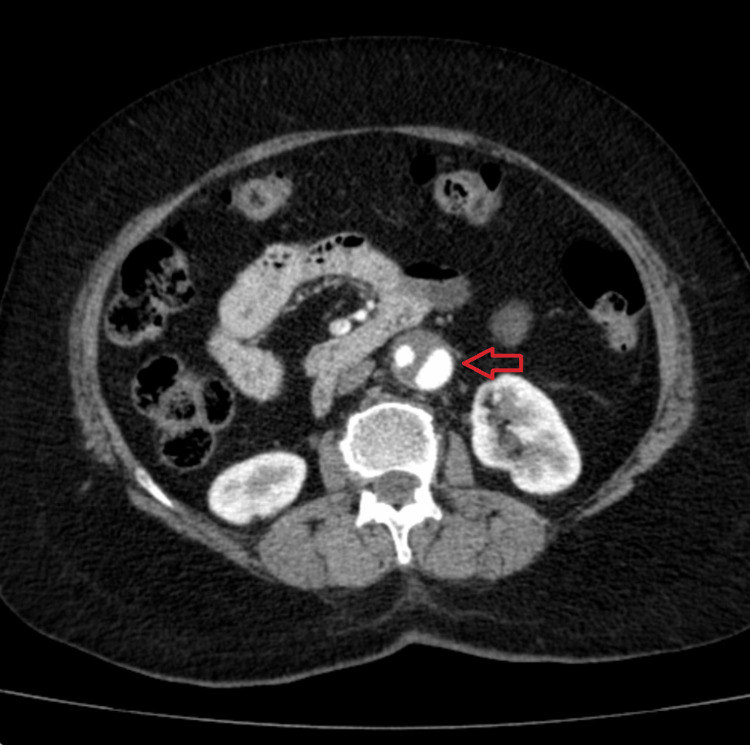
Axial contrast-enhanced CTA demonstrating an infrarenal abdominal aortic dissection The axial image demonstrates an intimal flap (red arrow) within the infrarenal aorta, resulting in a double-lumen appearance characteristic of aortic dissection, with clear differentiation between the true and false lumens. CTA, CT angiography
